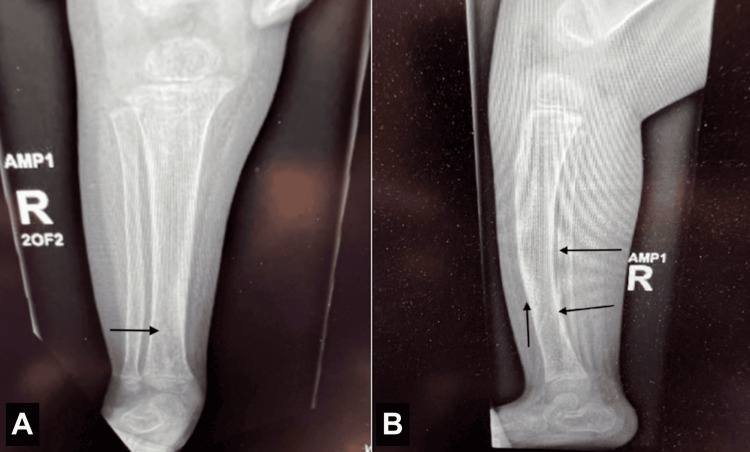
X-ray of the right tibia (A) axial and (B) sagittal views. Arrows refer to a nondisplaced distal tibial toddler's fracture along with mild osteopenia, mild bowing, and diaphyseal thickening.
